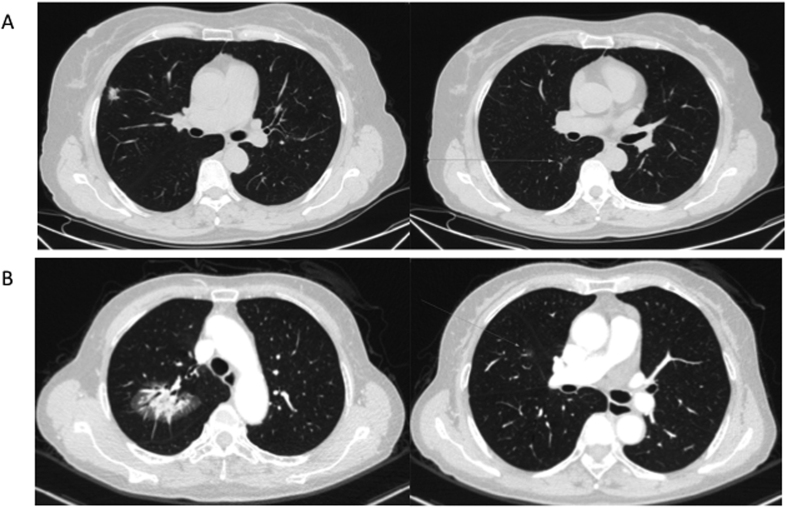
CT scan images for two MPLC patients. (A) Images of Patient I. Left, CT scan of upper right lobi pulmonis; and right, CT scan of lower right lobi pulmonis. (B) Images of Patient II. Left, CT scan of upper right lobi pulmonis; and right, CT scan of middle right lobi pulmonis.
